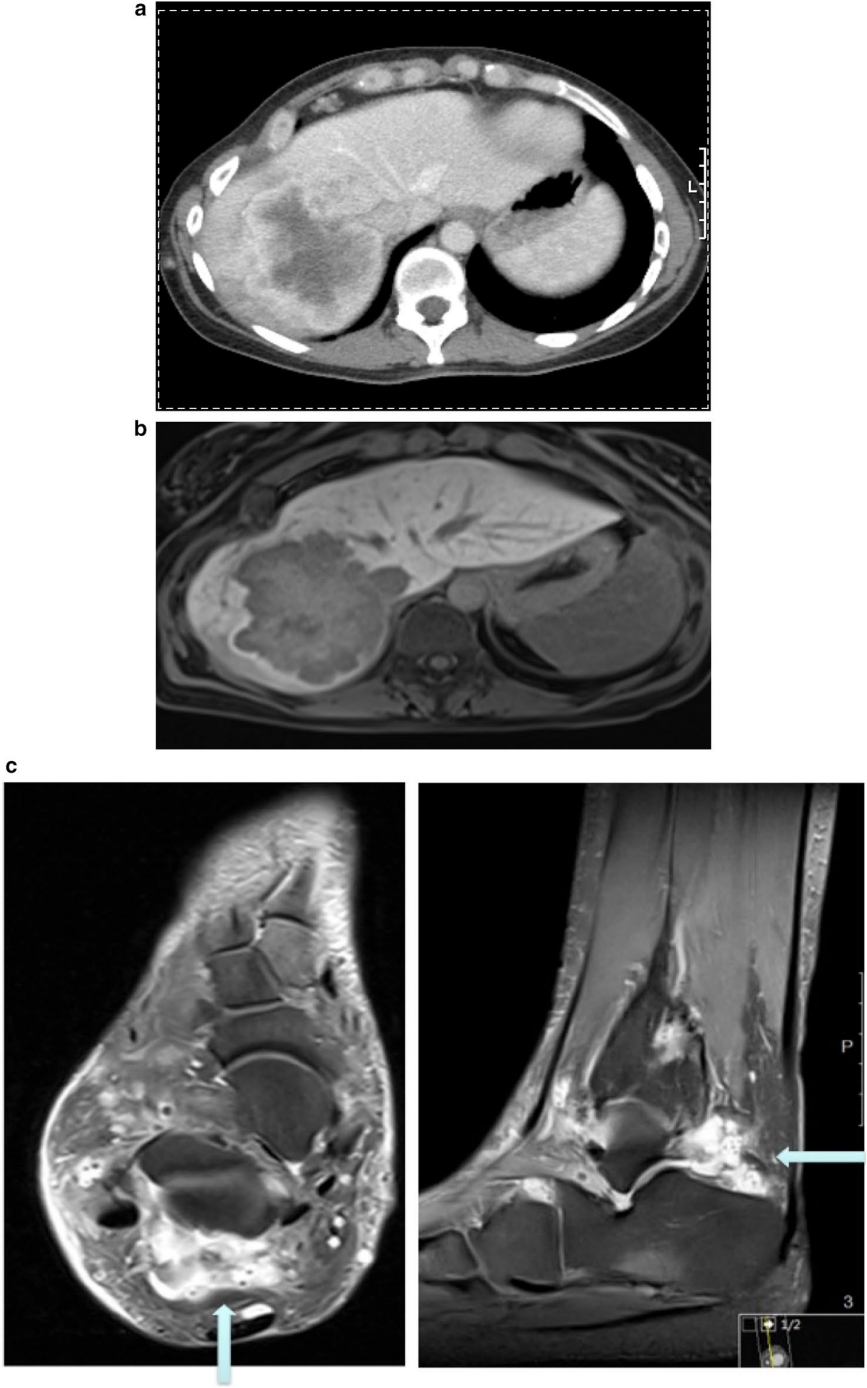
Preoperative imaging studies. a Computed tomography of liver tumor showed a 9 cm mass in the right lobe of liver; b Gadoxetic acid (primovist)-enhanced MRI demonstrated a lesion in liver segments VII and VIII, peripherally hypointense compared to surrounding *liver in T2w*, centrally hyperintense. A strong arterial hypervascular enhancement was demonstrated after primovist administration and there was a failure to enhance in the hepatobiliary phase. The tumor has contact with the inferior vena cava and some mm distance from middle liver vein. T1 fat saturated post gadoxetic acid. Hepatobiiliary phase (20 min post injection). c Soft tissue hemangioma (MRI). Hemangioma dorsal to upper ankle joint, PDw fat saturation (proton density weighted, fat saturated MRI)
